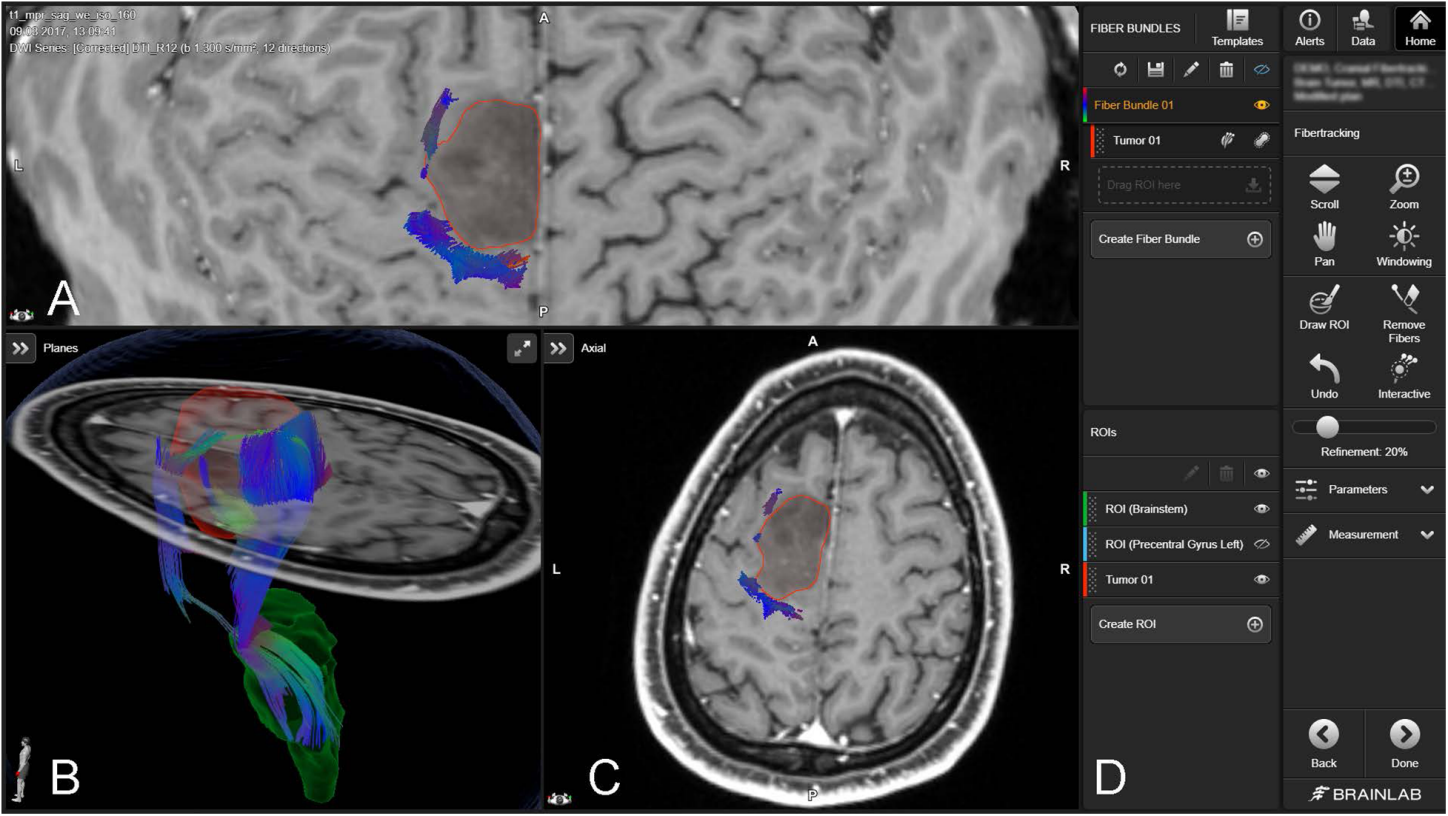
Fiber tracking with brainlab elements fibertracking (45). The figure includes a 3D rendering and cross-sectional MRI views [axial (A,C), and coronal (B)] of the brain, with a tumor outlined in red. The tractography data visualize the fiber bundles (depicted in various colors), indicating their spatial relationship to the tumor. The software interface on the right (D) provides functionalities for manipulating views, creating regions of interest (ROIs), and tracking fibers.
